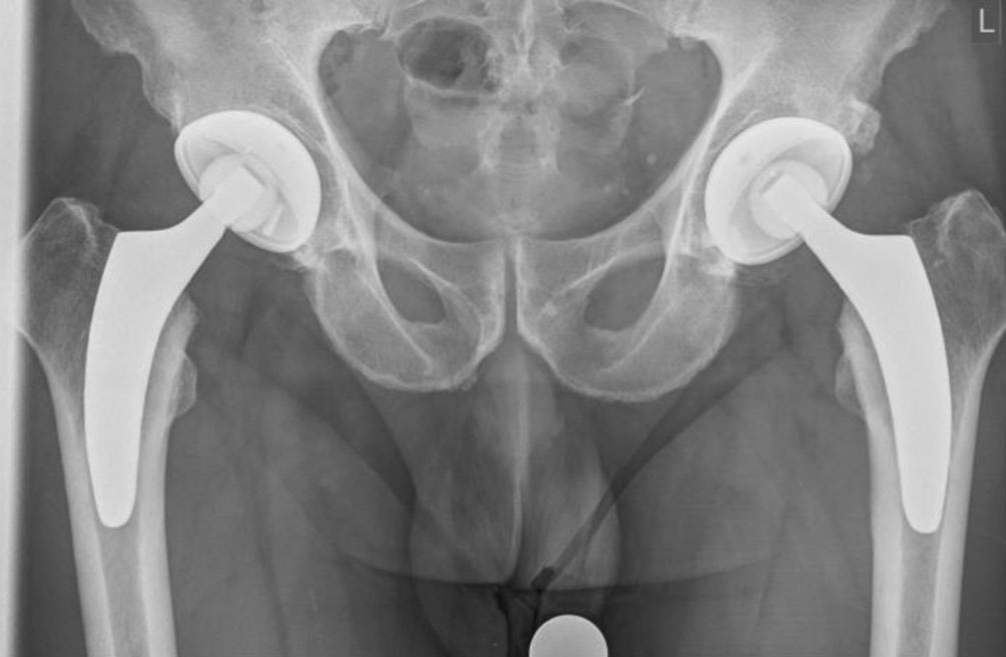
Postoperative radiograph of a one-stage bilateral calcar-guided short-stem THA
